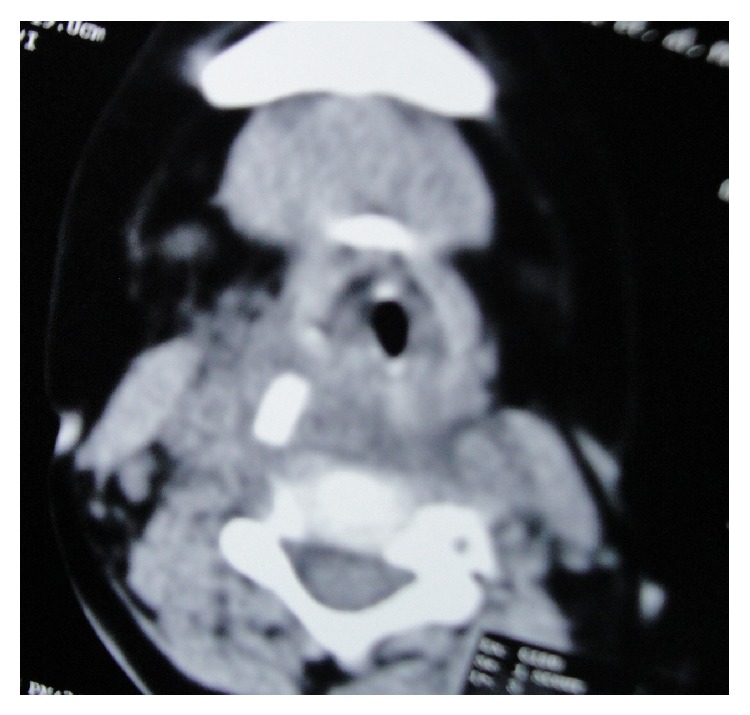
CT scan showing the lowermost part of the toothbrush in the retropharyngeal space of the hypopharynx.
